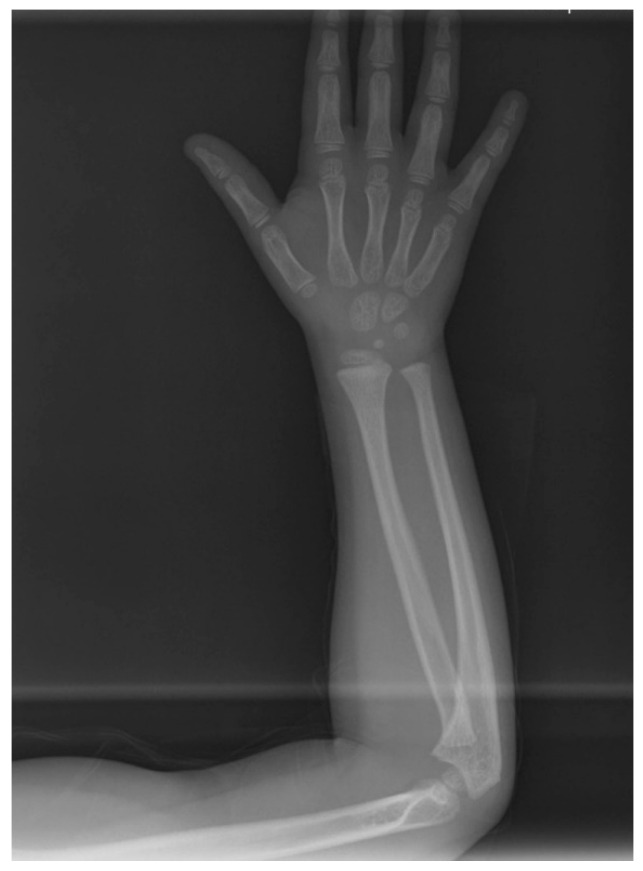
Hand-wrist and long bone radiographic presentation of the daughter exhibiting no osseous defects.
